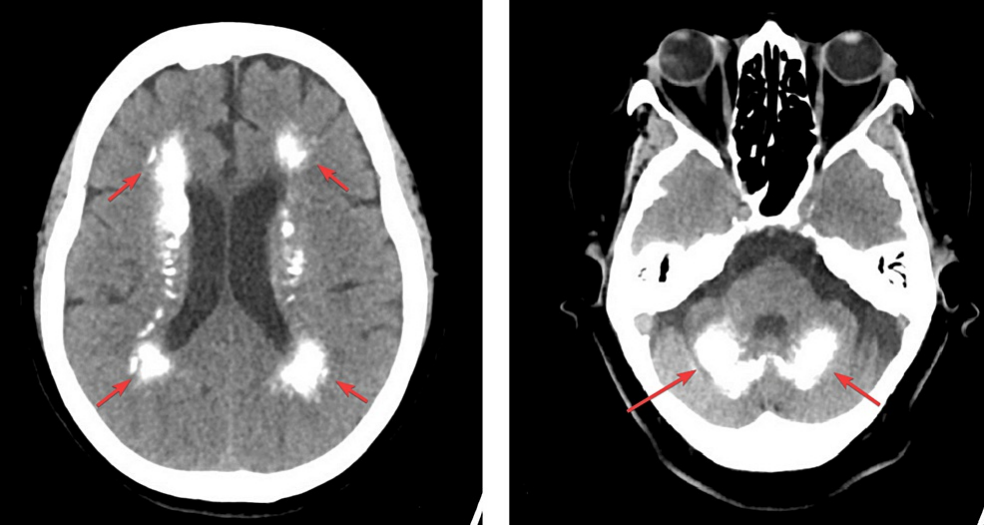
CT scans of the head without contrast showing extensive diffuse calcification involving the basal ganglia, centrum semiovale, posterior occipital lobes, and the cerebellum. The occipital lobes’ calcification involves the cortex.
